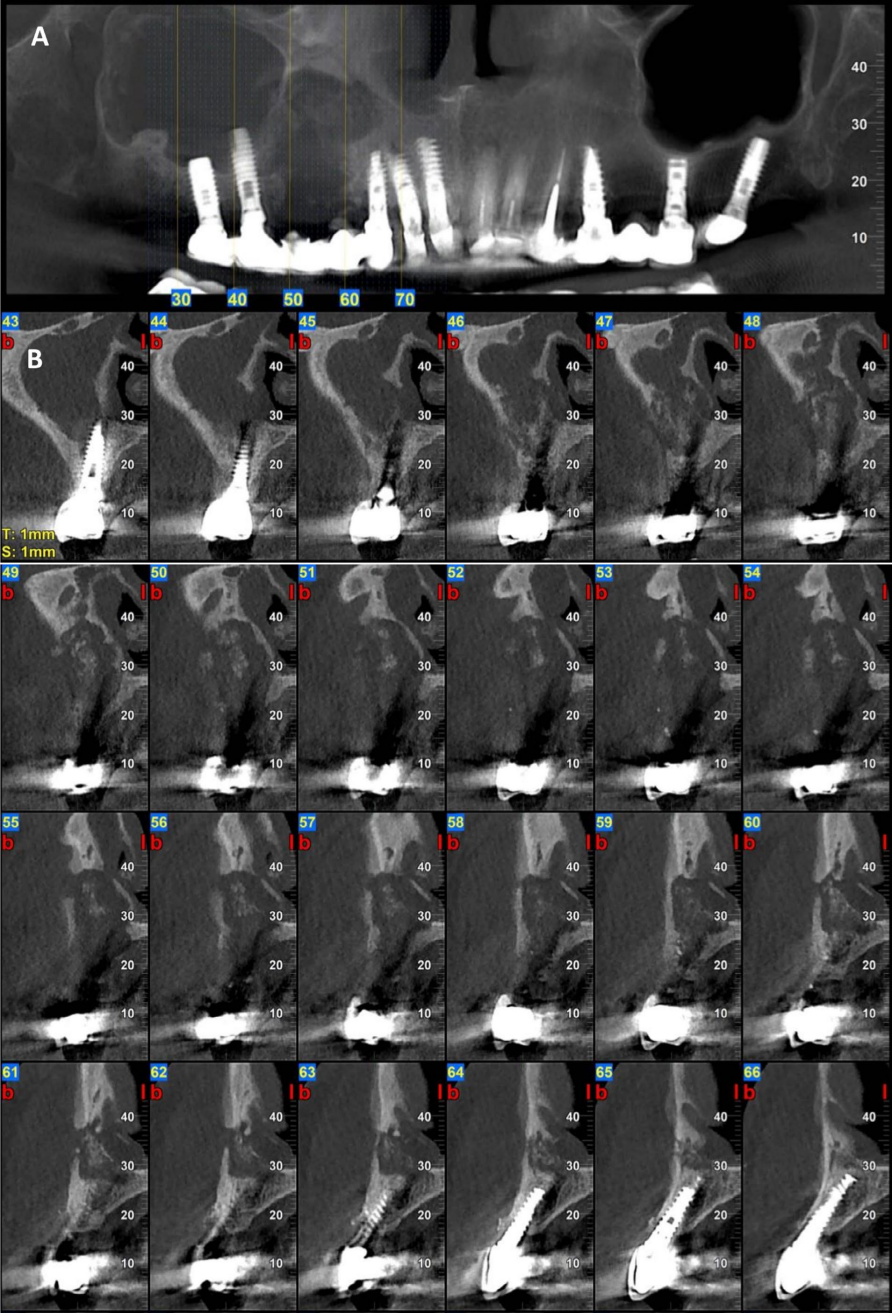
Cone‐beam CT imaging at initial presentation—Case 3. (A) Panoramic reconstruction from cone‐beam computed tomography (CBCT) reveals a well‐defined osteolytic lesion involving the right posterior maxilla, extending between dental implants and into the maxillary sinus. (B) Axial CBCT view demonstrating pronounced osteolysis with visible sequestrum.
